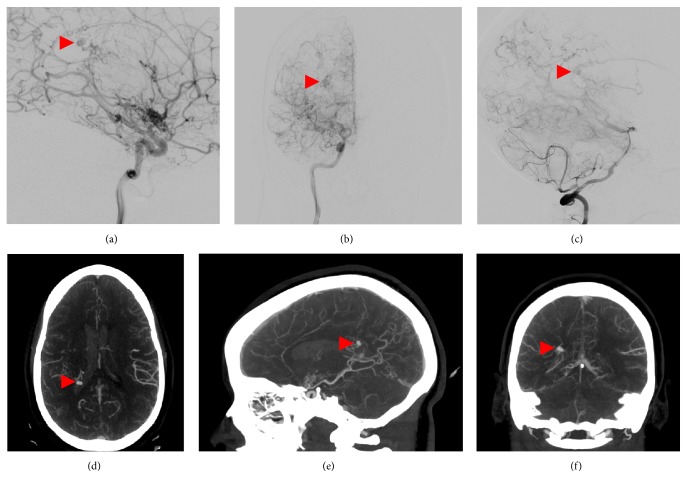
Cerebral angiography, (a) lateral and (b) AP projections of a right internal carotid artery injection and (c) lateral projection of a right vertebral artery injection, shows an aneurysm (arrowhead) arising from the medial posterior choroidal artery branch of the right posterior cerebral artery, with stagnation of contrast in the late arterial phase. Preoperative CTA, (d) axial, (e) sagittal, and (f) coronal views, shows a 5 × 4 × 3 mm periventricular aneurysm (arrowhead), projecting into the atrium of the right lateral ventricle with multiple adjacent collateral vessels.
